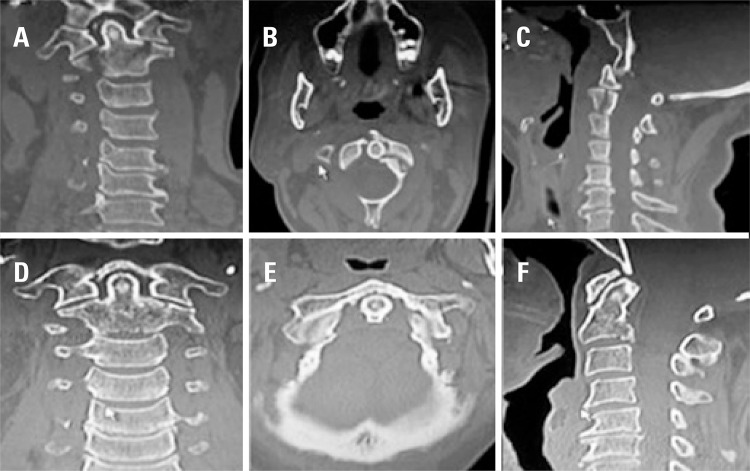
Combined anterior arch of C1 and C2 body fractures - conservative treatment. Line 1: computed tomography images showing the fractures before treatment. (A) coronal, (B) axial, (C) sagittal sections. Line 2: fractures are consolidated after eight weeks of conservative treatment. (D) coronal, (E) axial and (F) sagittal sections
